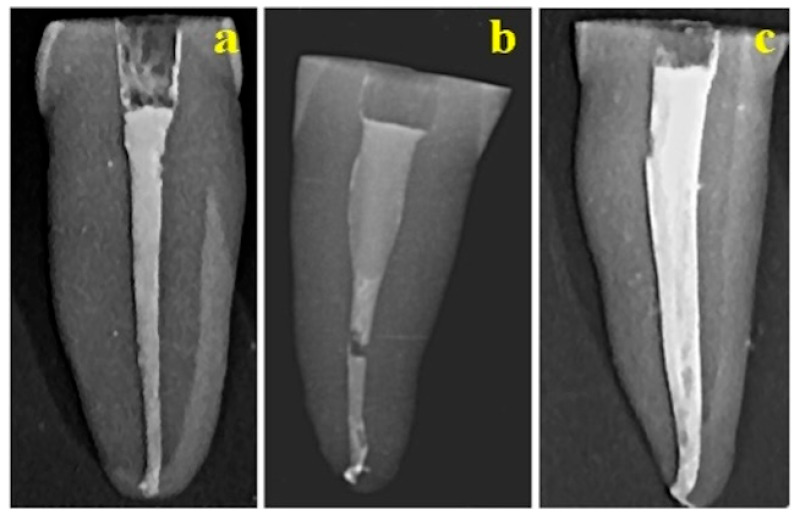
Radiographs of root canal fillings performed using the following methods: (a) SC technique, (b) WVC technique, and (c) LC technique.
